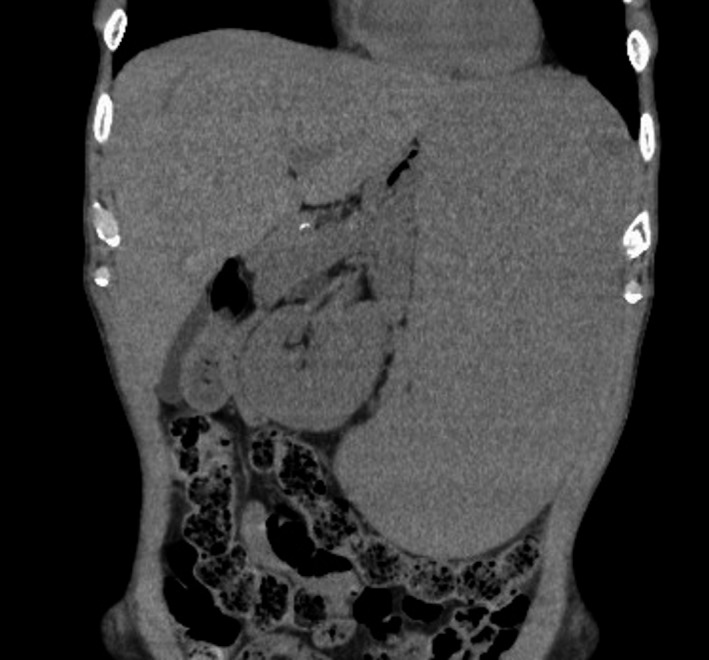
Computed tomography showed massive splenomegaly
